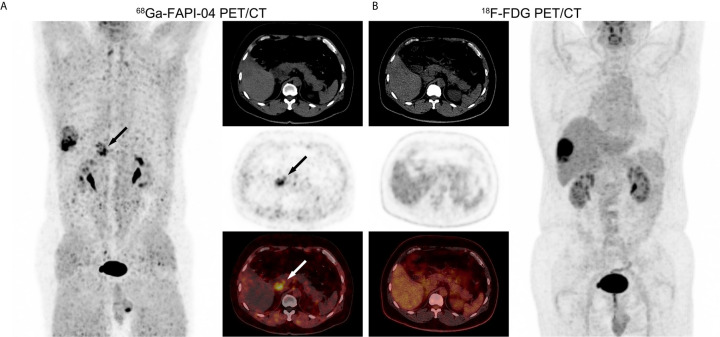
PET/CT images in a 53-year-old male patient with recurrent HCC and extensive peritoneal dissemination. (A) In ^68^Ga-FAPI-04 PET/CT, a small metastatic lesion confirmed by pathology showed elevated uptake (black and white arrows, SUV_max_ = 4.72, TBR = 11). (B)
